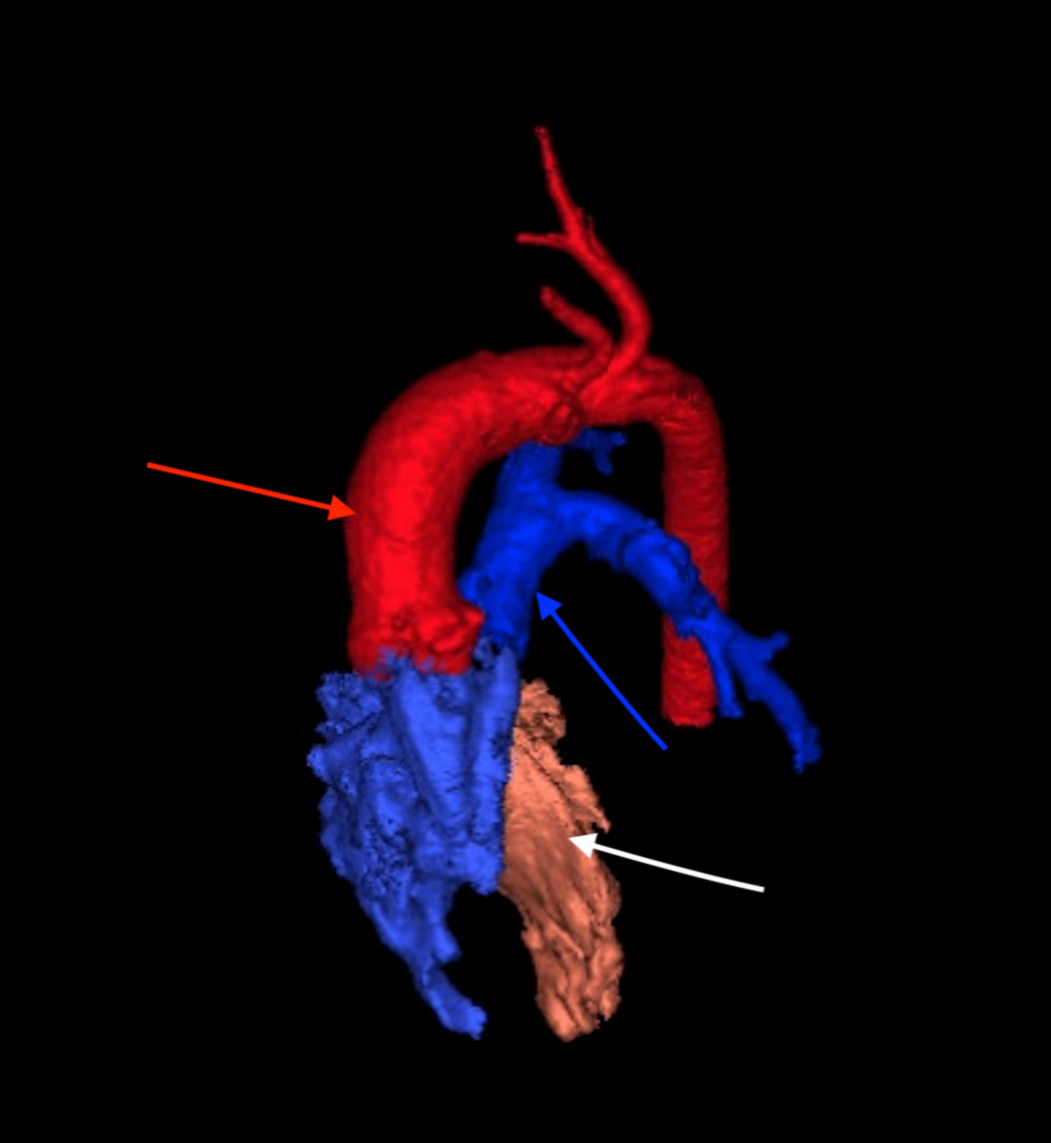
Three-dimensional computed tomography reconstruction demonstrating double outlet right ventricle with D-malposed great arteries (anterior superior view) The red arrow indicates the aorta, which arises anteriorly and rightward from the right ventricle. The blue arrow marks the malposed pulmonary artery, arising posteriorly and leftward. The white arrow identifies the right ventricle, from which both great arteries originate.
